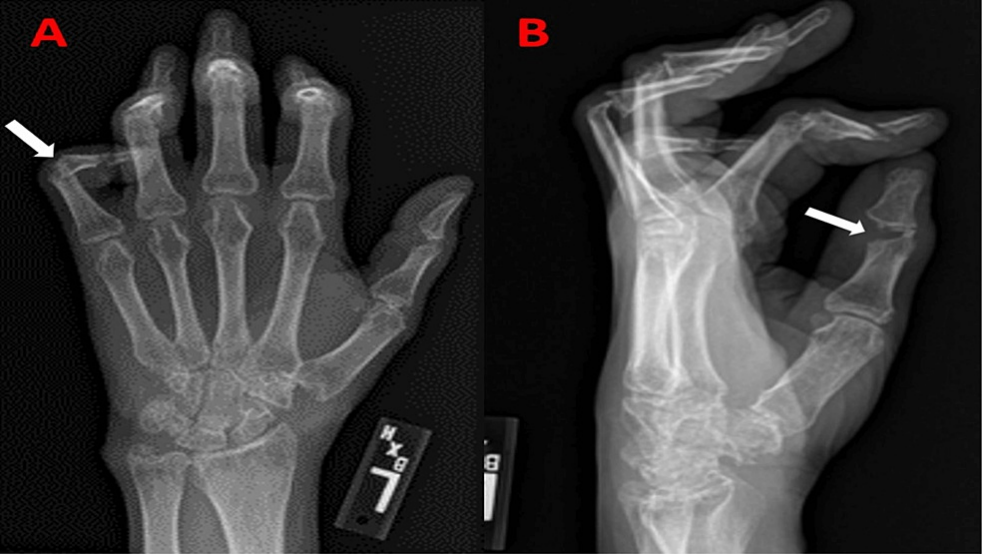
X-ray left hand posteroanterior (A) and lateral view (B) demonstrating destructive interphalangeal joints (white arrows) with loss of bony substance around the interphalangeal joint.
